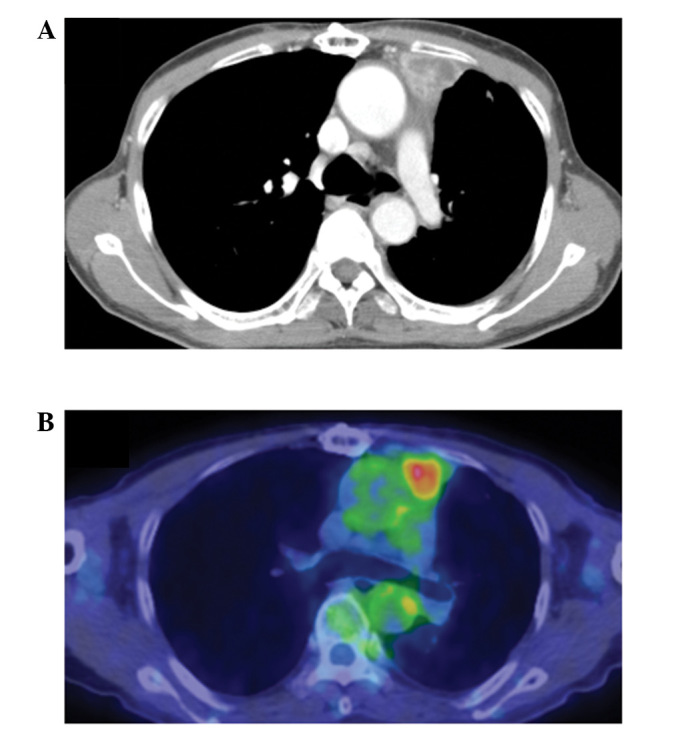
Imaging observations. (A) CT revealing an anterior mediastinal tumor with a maximum size of ∼3 cm. (B) PET/CT scan revealing abnormal uptake of ^18^F-fluorodeoxyglucose in the tumor. CT, computed tomography; PET, positron emission tomography.
